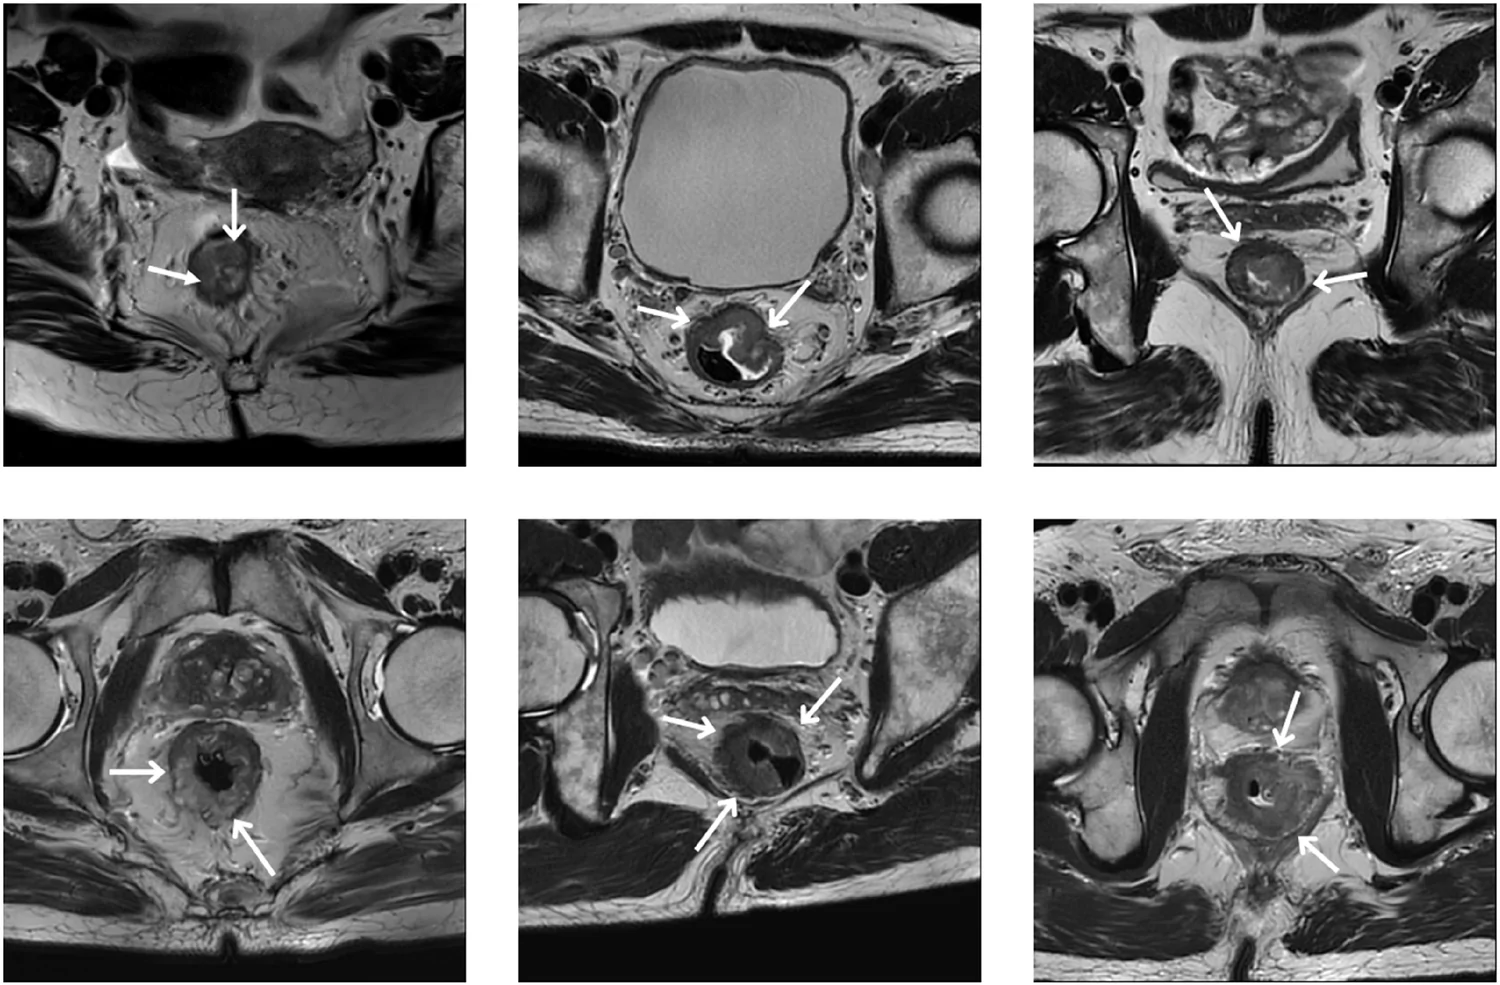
Representative T2-weighted imaging (T2WI) examples of rectal cancer. The first and second rows show tumors pathologically confirmed as T2 and T3 stages, respectively
